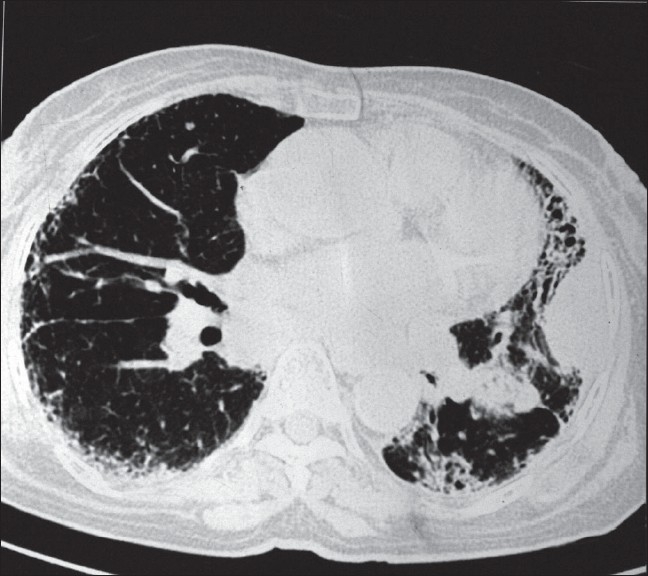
HRCT thorax showing honeycombing with bilateral reticular lesions and a left-sided pleural-based mass
